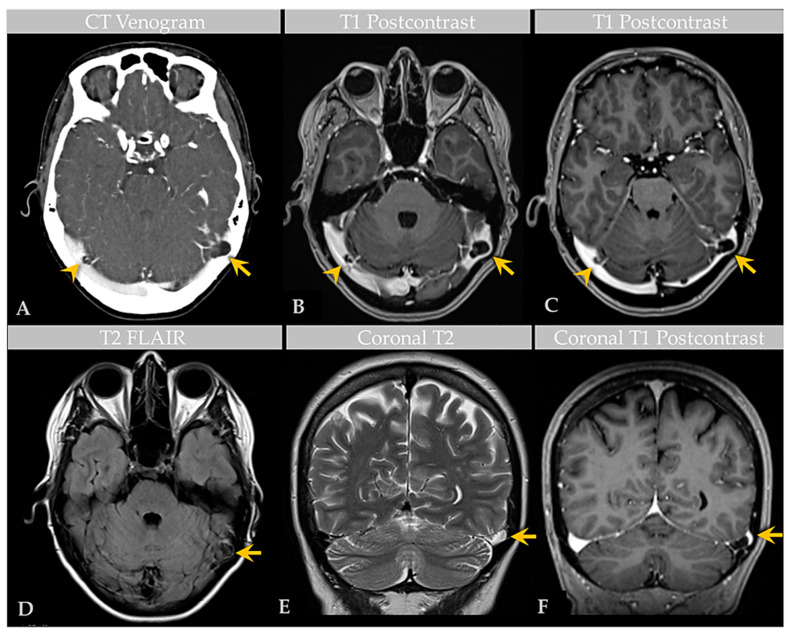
Case 1: Imaging of an individual with multiple GAGs. CT venogram (A) shows GAGs indenting the lateral aspect of bilateral TS (arrow, arrowhead). Postcontrast T1-weighted MRI images (B,C) demonstrate bilateral TS GAGs, measuring 1.6 cm on the left (arrow) and 1.1 cm on the right (arrowhead). The GAG is associated with focal severe narrowing of the lateral left TS (arrow, (C)). Axial T2-weighted FLAIR image (D) demonstrates heterogeneous signal within the GAG (arrow), likely reflecting internal soft tissue elements with CSF flow turbulence. Coronal T2-weighted (E) and postcontrast T1-weighted (F) images show high-grade TS luminal narrowing by the GAG (arrows). An additional SSS GAG is not depicted.
